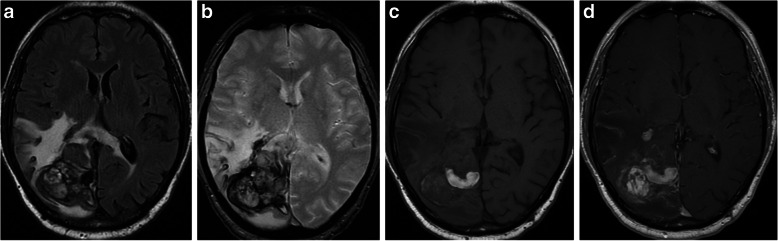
Case 5. A 49-year-old man presented with intracranial hemorrhage related to a right occipital AVM. The lesion was partially treated with embolization. A nidal remnant was further treated with SRS with a marginal dose of 18 Grays. Thirteen years post SRS, the patient presented hemiparesis. a Flair, (b) T2*, (c) T1 and d) T1 contrast enhanced MR images show a large heterogeneous cavernoma-like lesion with extensive edema. Surgery was judged as too risky and the lesion finally stabilized under steroid therapy
